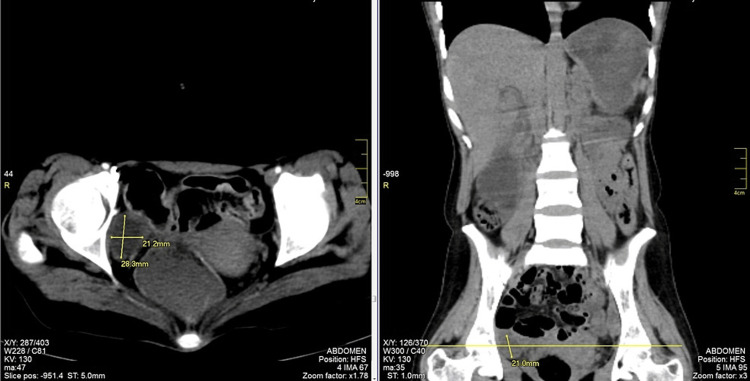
The CT scan revealed a right adnexal thin-walled cyst, measuring 2.8x2.1 cm. "HFS" in MRI stands for "Head First Supine," indicating a patient positioning with the head entering the scanner first while lying on their back. AOM, aortomesenteric
